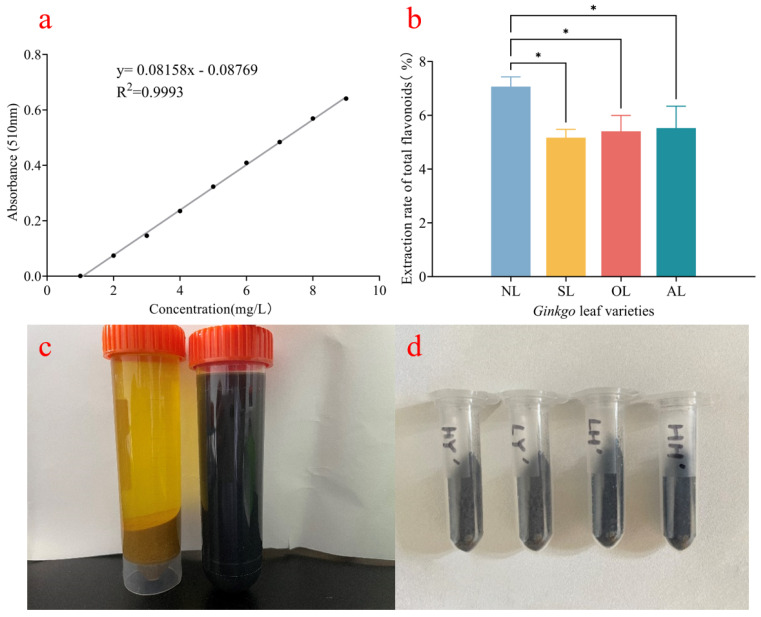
Green synthesized Fe_3_O_4_NPs. (a) Rutin standard curve; (b) The data in the figure are presented as mean ± standard deviation. * indicates significant differences between treatments (*p* < 0.05). *G. biloba* leaf types are denoted as follows: NLs for fallen yellow leaves, SLs for unshed yellow leaves, OLs for mixed yellow leaves, and ALs for green leaves. (c) The right side is the ginkgo extract solution, and the left side is the green synthesized nanoscale iron preparation solution; (d) Nanoscale iron extracted from different ginkgo leaves using ultrasonic-assisted enzymatic extraction method.
